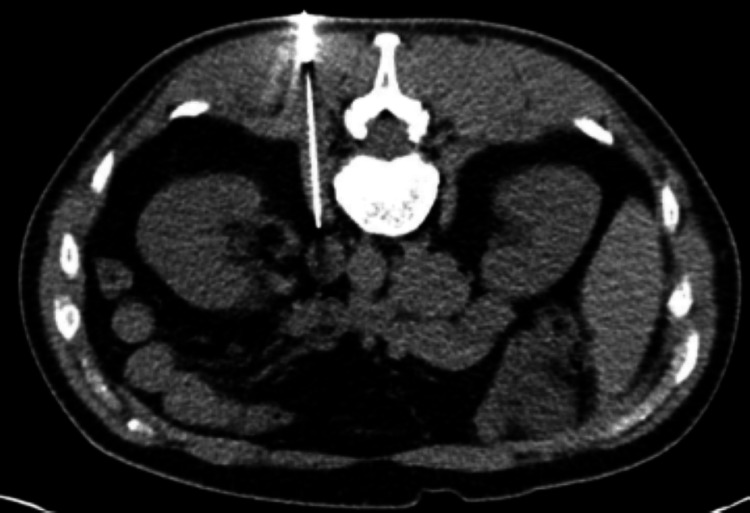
Computed tomography (CT)-guided core needle biopsy of a retroperitoneal lymph node. The figure demonstrates needle placement under real-time CT guidance for tissue sampling, which subsequently confirmed metastatic pulmonary adenocarcinoma.
